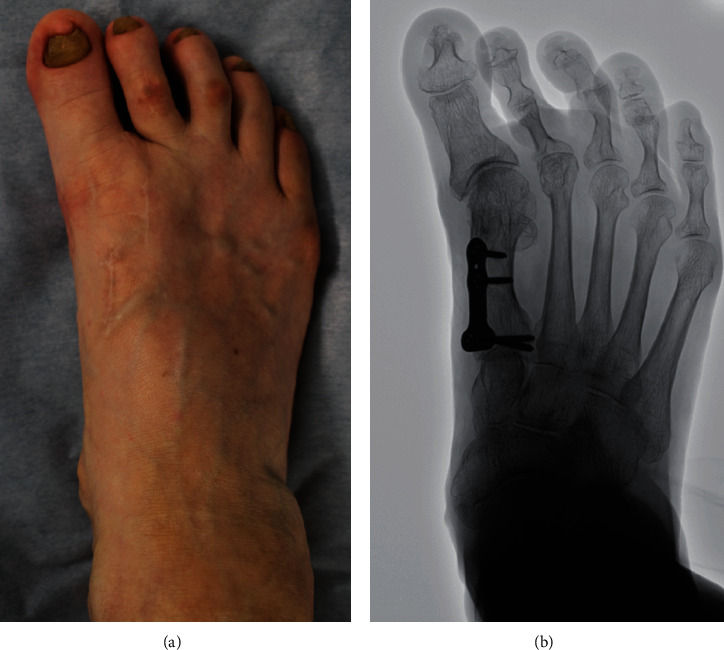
Preoperative appearance (a) and plain radiograph in the standing position (b). The hallux valgus angle was -24° and the intermetatarsal angle 0°.
